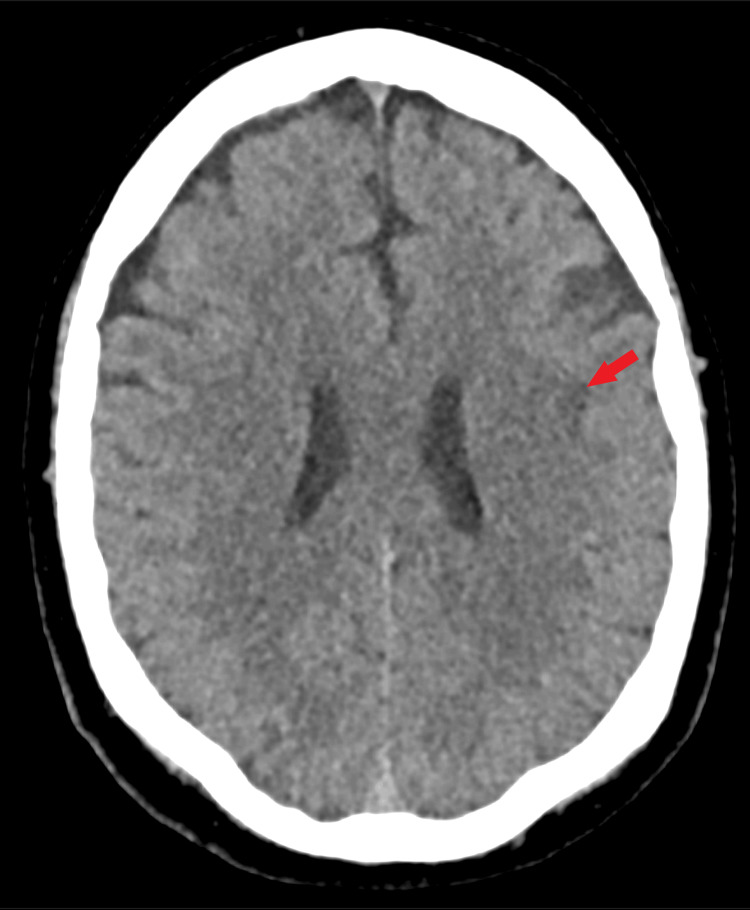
Axial head computed tomography (CT) imaging without contrast demonstrates a hypodensity in the subcortical white matter in the posterior aspect of the left frontal lobe (red arrow).
